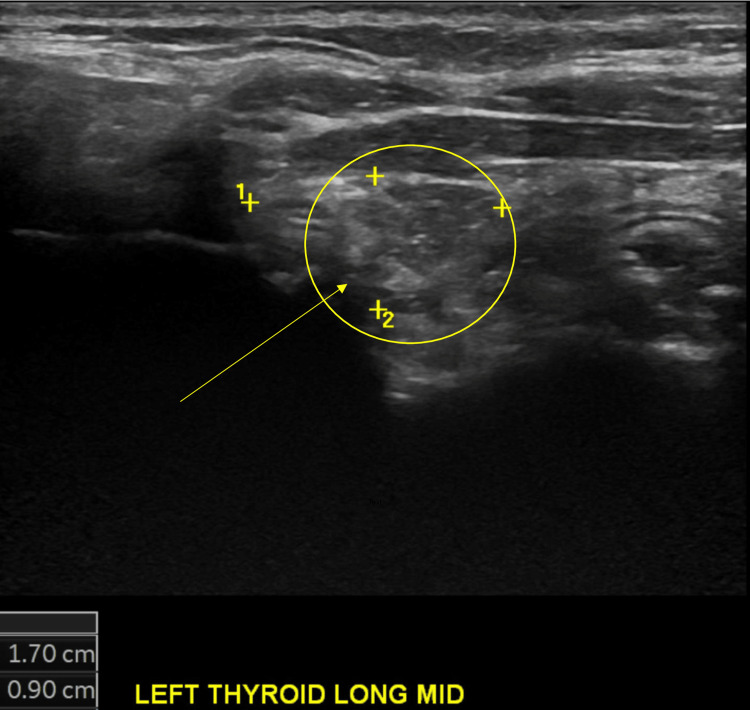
Ultrasound of the left thyroid. The circle contains multiple left thyroid nodules. The arrow is pointing to the largest left thyroid nodule.
